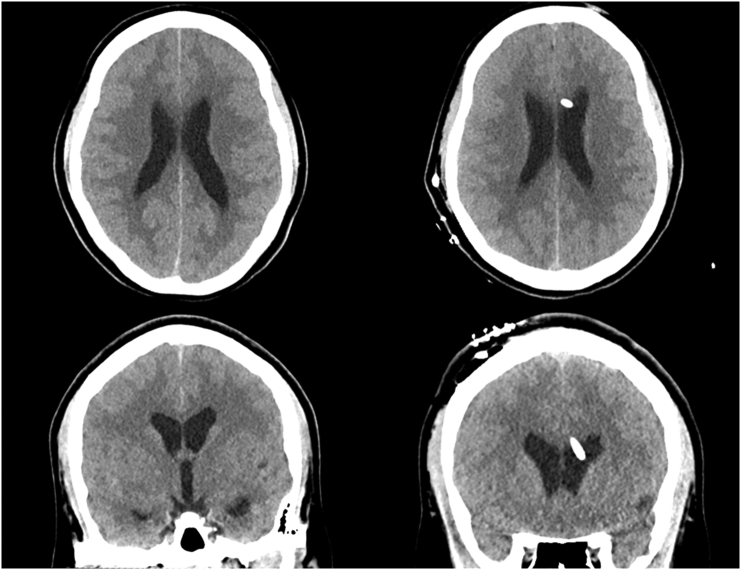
Preoperative vs postoperative head computed tomography (CT) axial scans showing (A) signs of preoperative obstructive hydrocephalus at initial presentation, and (B) the position of a ventriculoperitoneal shunt (VPS) in the eft lateral ventricle.
